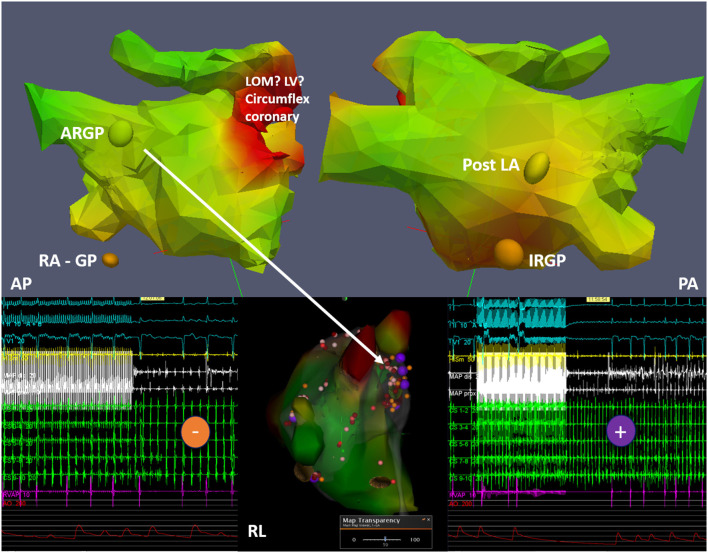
Example of ^123I^-MIBG imaged-guided ganglionated plexus (GP) modulation in a patient with paroxysmal atrial fibrillation. The top panels depict three-dimensional (3D) reconstructions from ^123I^-MIBG nuclear image merged with contrast computed tomography imaging with discrete uptake sites of ^123I^-MIBG demonstrated as colored spheres (ARGP, anterior right GP; IRGP, inferior right GP; RA GP, right atrial GP; post LA GP, located in the posterior LA wall). The middle lower panel shows the integration into the electroanatomical mapping system with the results of the high-frequency stimulation (HFS) shown as colored tags (purple, positive HFS effect with temporary AV block; orange, negative HFS effect). In addition to radiofrequency ablation at positive HFS sites, conventional isolation of the pulmonary veins is subsequently performed. Other abbreviations: AP, antero-posterior projection; PA, postero-anterior projection; RL, right lateral projection; LV, left ventricle; LOM, ligament of Marshall.
